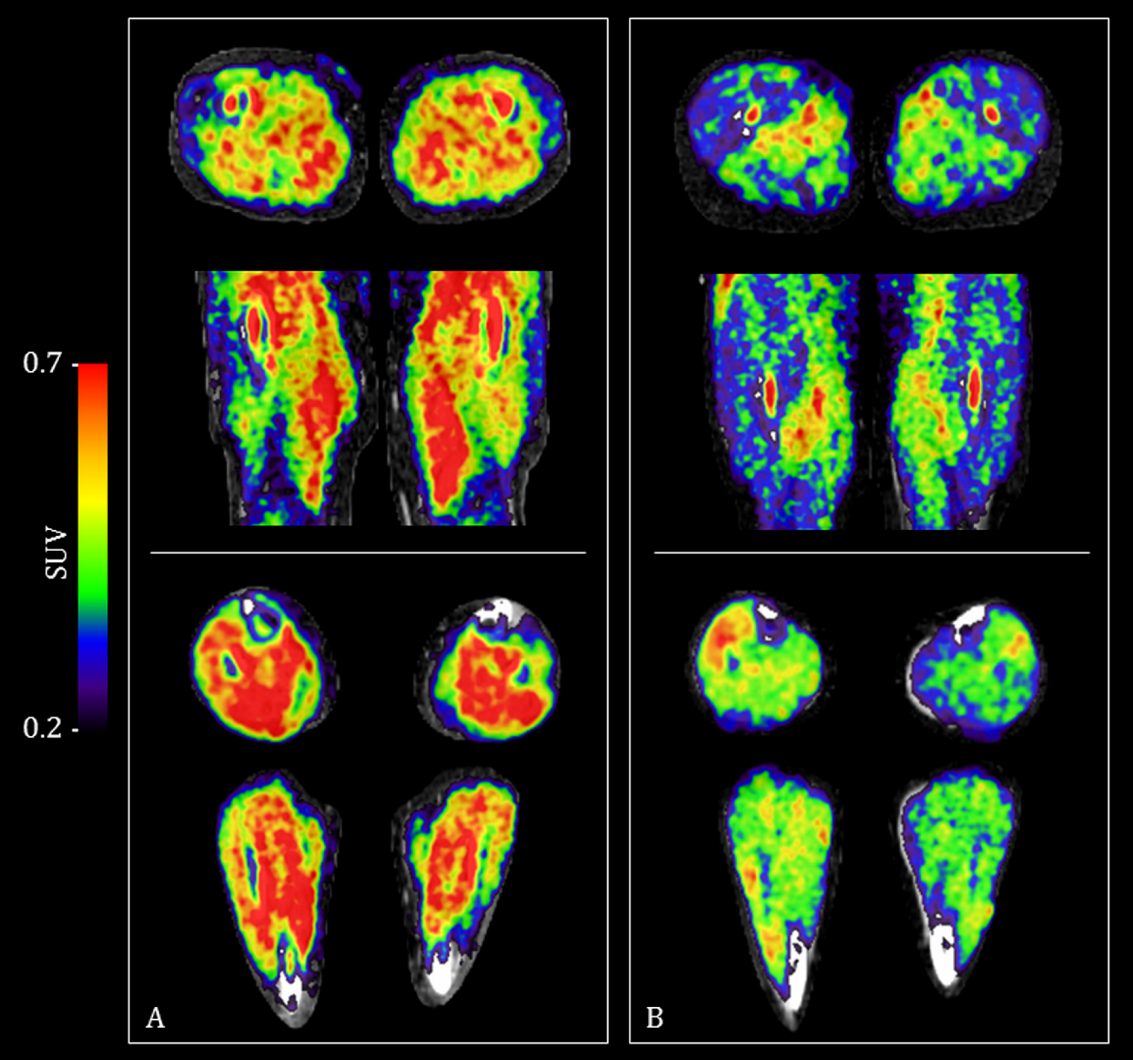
(18F)florbetapir PET/CT images showing differences in uptake between a participant with inclusion body myositis (panel A) and one with polymyositis (panel B). Increasing SUVs (red) indicate increased tracer uptake. [18F]Florbetapir PET images overlay spatially aligned CT images. Top of each panel depicts axial and coronal slices though the thigh. Bottom of each panel depicts axial and coronal slices though the calf. Each image is centred on the middle of the defined region of interest. PET, positron emission tomography; SUVs, standardised uptake values.
